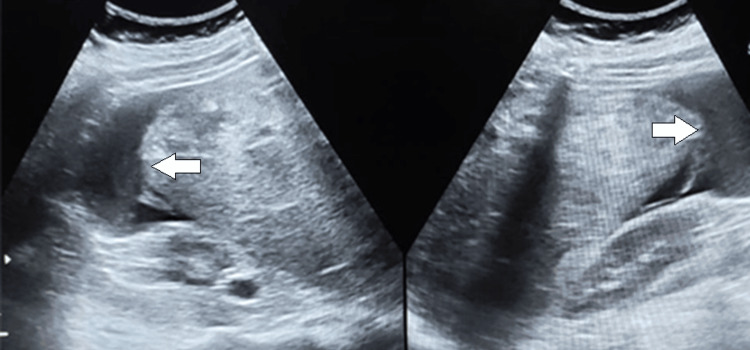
Ultrasonographic examination of the abdomen shows nonspecific heterogeneous hepatic parenchyma and perihepatic free fluid effusion (arrow).
